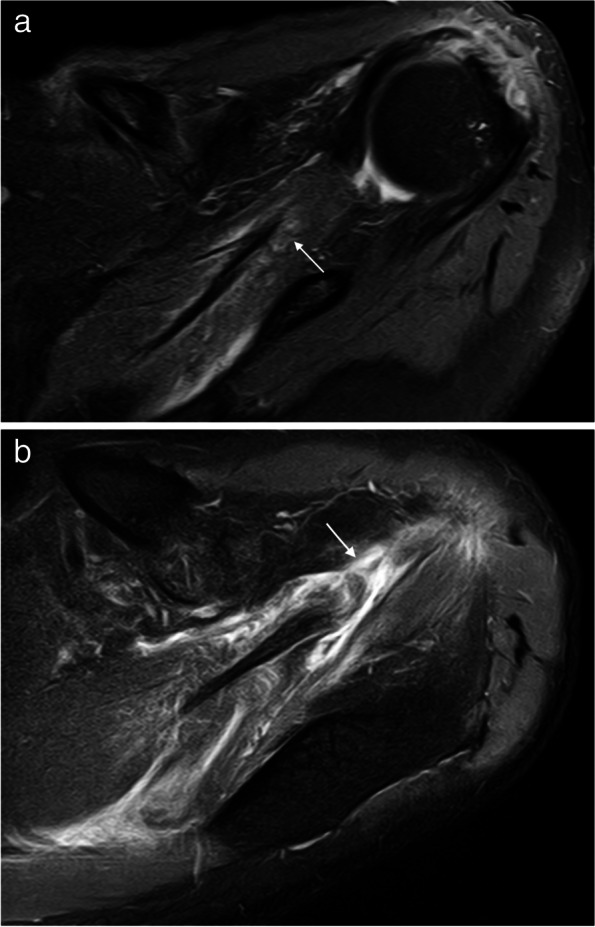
Supraspinatus myotendinous junction tears. a, b Axial fat-suppressed PD-weighted MRI. a Shows edema of the anterior muscle belly of the SS and a partial tear of the myotendinous junction (arrow). b Reveals a full thickness retracted tear of the myotendinous junction of the anterior belly of the SS (arrow)
